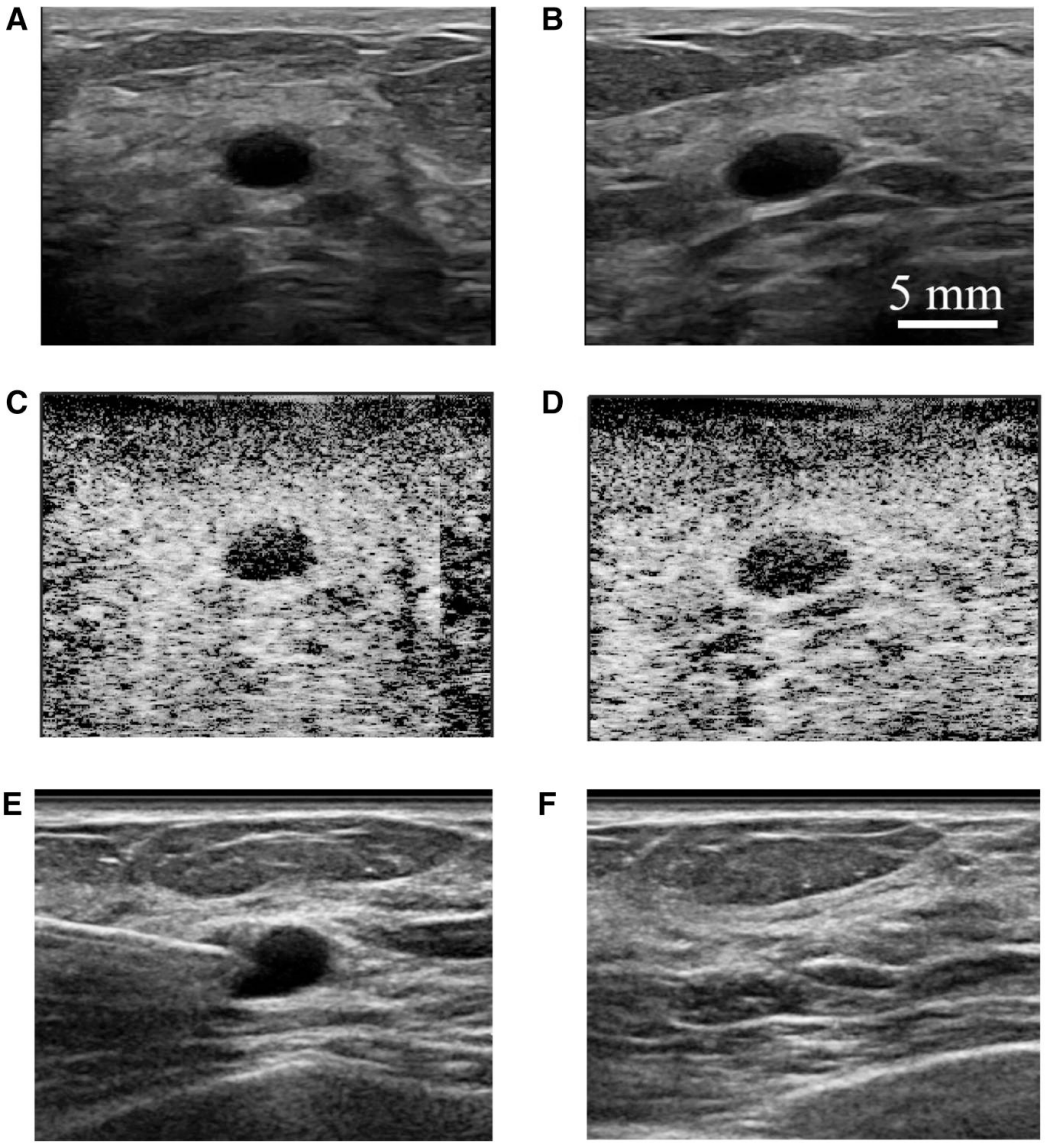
A 60-year-old woman was evaluated on follow-up imaging of a left breast sonographic mass, which had slightly increased in size compared to a prior ultrasound. Pairs of B-mode ultrasound static images show a 6 mm mass seen in the (A) antiradial and (B) radial projections at 8 mm depth from the ultrasound probe. (C) Antiradial and (D) radial SLSC images of the same finding show darker (or less coherent) lesion content relative to the surrounding tissue. Six radiologists characterized the finding as fluid based on B-mode and SLSC ultrasound images. Five of the 6 radiologists drew ROIs resulting in gCNR > 0.76 for the SLSC image, with a gCNR of 0.75 produced by 1 radiologist. Ultrasound-guided aspiration was performed with the (E) pre- and (F) post-aspiration image demonstrating complete aspiration of the finding yielding benign-appearing fluid, consistent with a benign complicated cyst.
